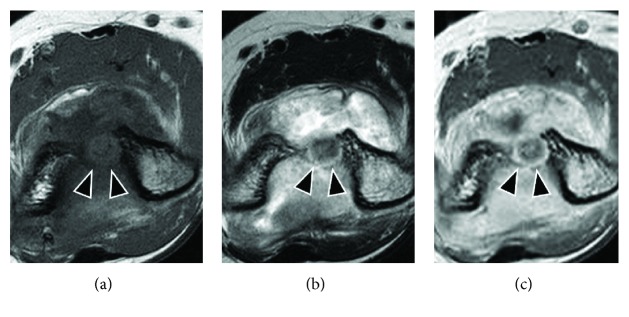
Axial T1-weighted (a), T2-weighted (b), and gadolinium-enhanced (c) images on preoperative magnetic resonance imaging. The round lesion shows slightly hyperintense signals compared to adjacent muscles on T1-weighted imaging and hyperintense to intermediate-intensity signals on T2-weighted sequences, with heterogeneous enhancement (arrowhead).
